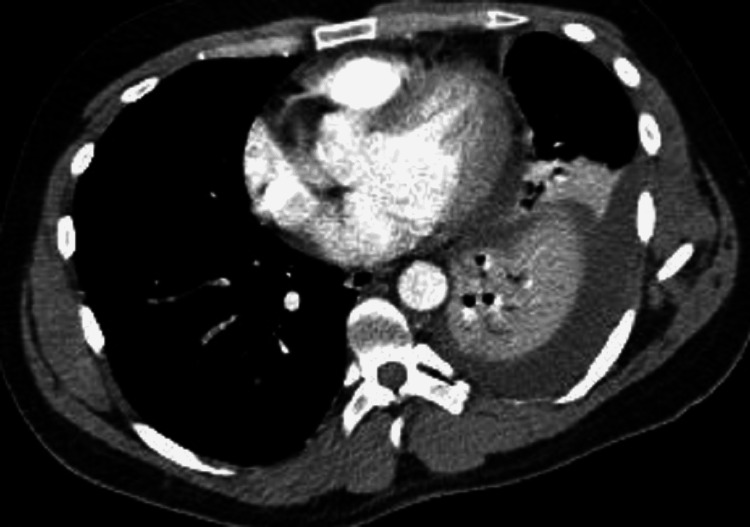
Subsequent computed tomography of the abdomen and pelvis showing left pleural effusion and compressive atelectasis on day nine.
